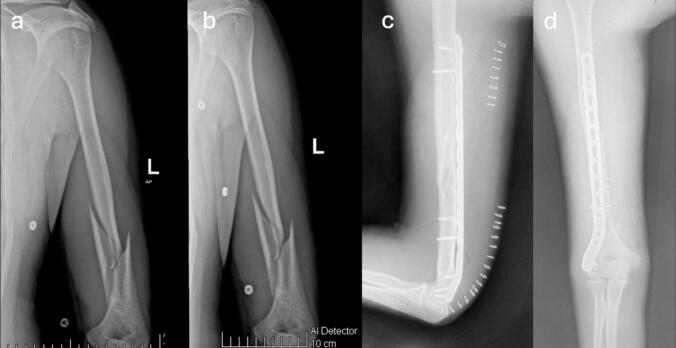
Case 3 – Female patient, 45 years old, mechanism of injury: fall from standing height. a-b) Preoperative AP and lateral radiographs showing AO 1.2.B.2-c fracture; c-d) Immediate postoperative AP and lateral radiographs; e-f) AP and lateral radiographs at 1-month follow-up.
